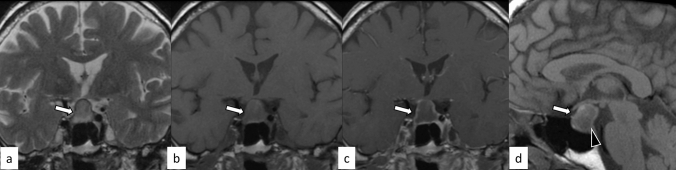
A 67-year-old man with plasmacytic/IgG4-related hypophysitis. An enlarged pituitary gland shows heterogeneous hyperintensity on T2-weighted coronal image (a, arrow) and hypointensity on T1-weighted coronal image (b, arrow) with peripheral enhancement and loss of central enhancement (c, arrow). The posterior pituitary T1-weighted bright spot is absent (d, black arrowhead)
